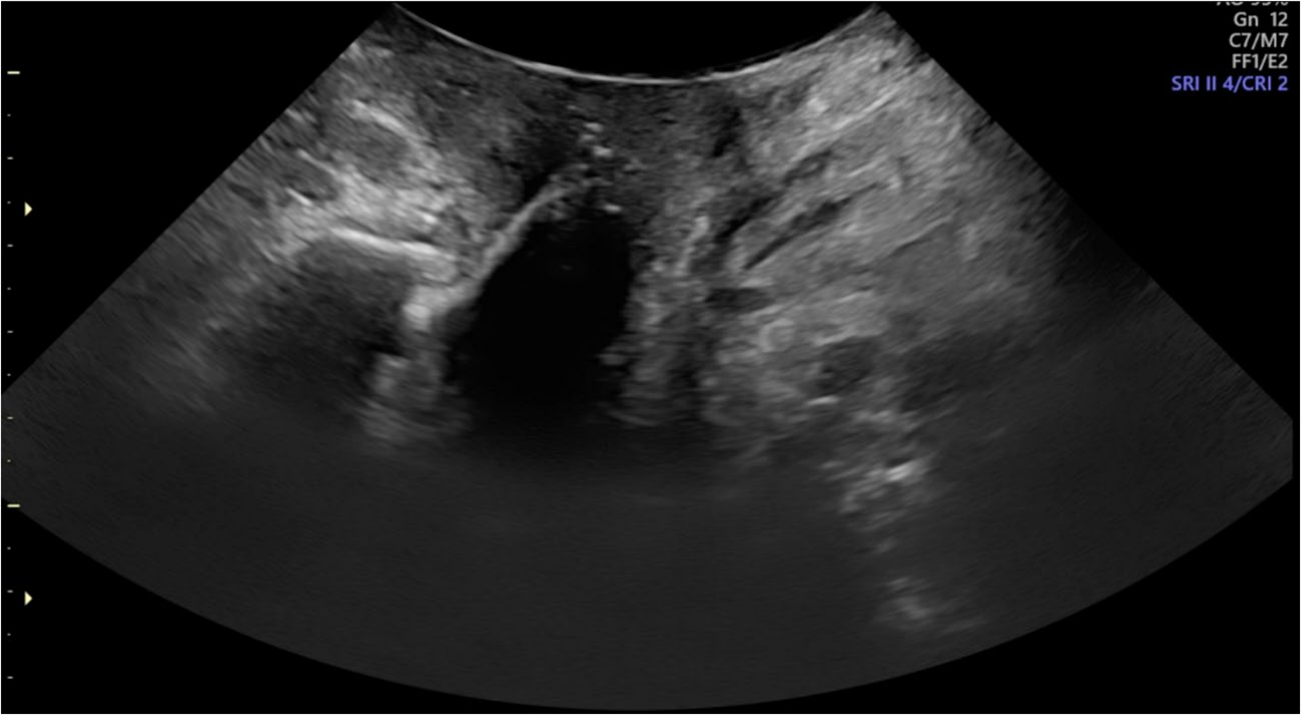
Transperineal ultrasound at rest with Valsalva demonstrating anterior compartment prolapse
